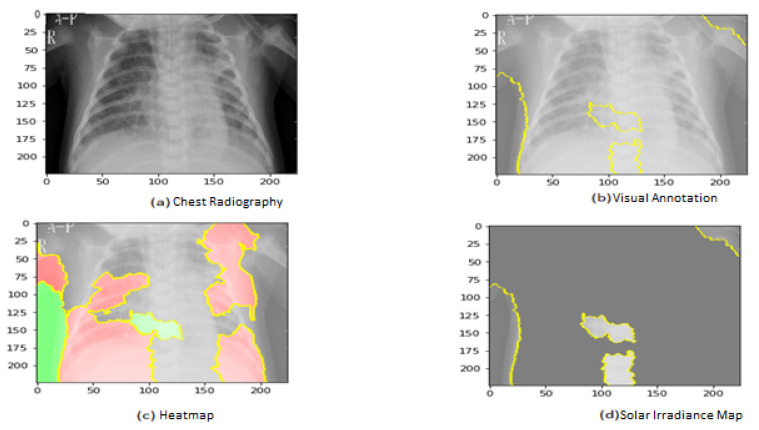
Explainability of X-ray images in the first dataset.
